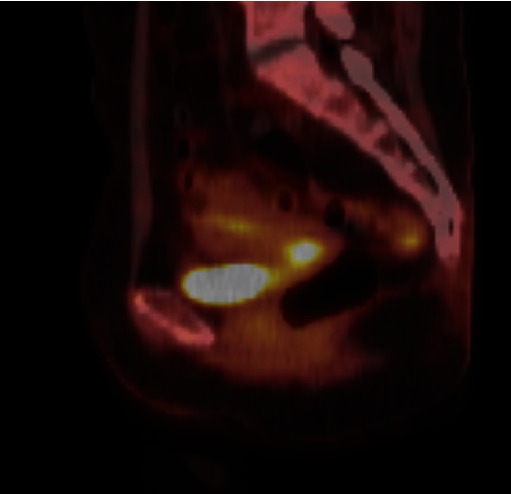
PET CT sagittal scan postradiation and presurgery with demonstration of a metabolically active mass within the cervix, consistent with the patient's known malignancy.
